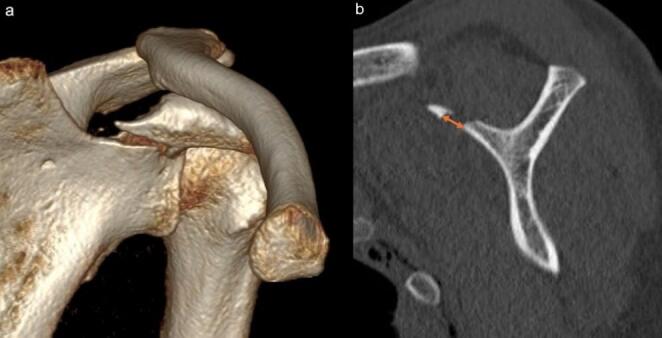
a An anteroinferolaterally displaced distal fragment in a coracoid process base fracture. b Measurement of the displacement gap at the medial edge of the fracture on a sagittal 3-D CT image
